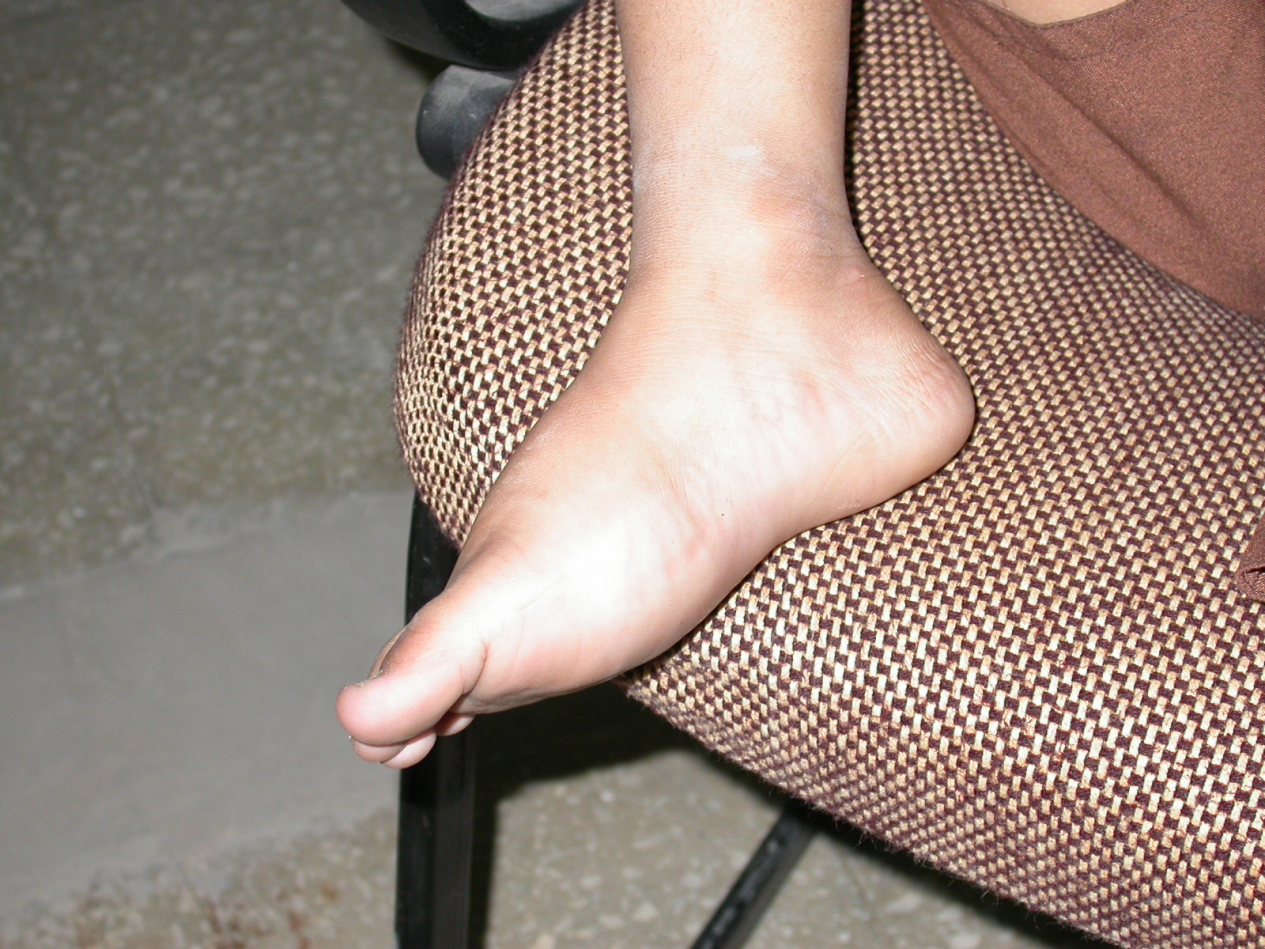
X-Ray (AP view) showing irregular destruction of medial cuneiform.
